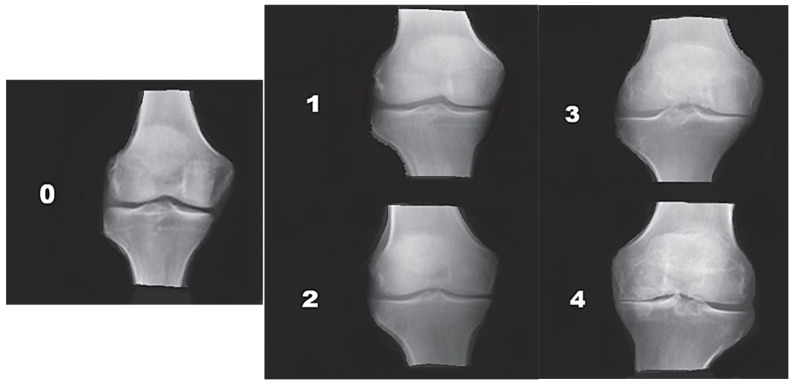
Kellgren–Lawrence (KL) radiographic grades of knee osteoarthritis. Representative anteroposterior knee radiographs demonstrating the five KL grades of osteoarthritis, from Grade 0 (no OA) to Grade 4 (severe OA). Grade 0: No radiographic features of osteoarthritis (normal joint appearance). Grade 1: Doubtful joint space narrowing with possible osteophytic lipping. Grade 2: Definite osteophyte formation with possible joint space narrowing. Grade 3: Multiple osteophytes, definite joint space narrowing, subchondral sclerosis, and potential bone contour deformity. Grade 4: Large osteophytes, severe joint space narrowing, pronounced subchondral sclerosis, and definite deformity of bone ends.
